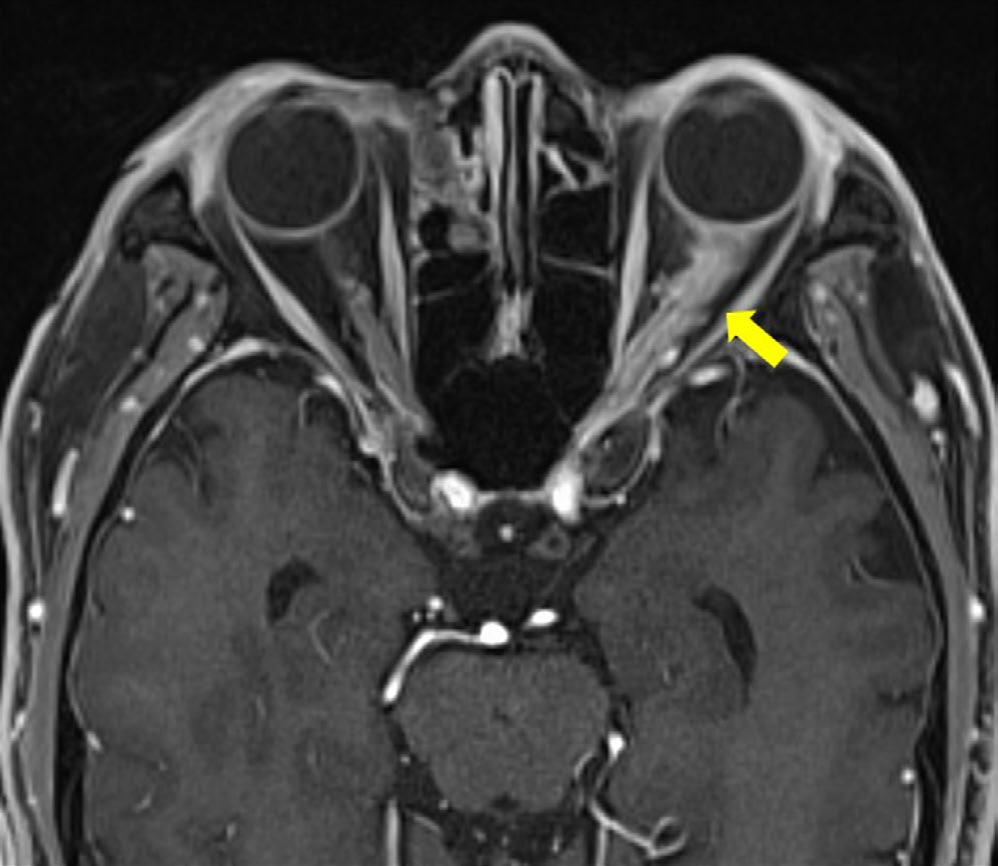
Findings of T1‐weighted orbital magnetic resonance imaging with contrast enhancement and fat suppression on admission. T1‐weighted orbital imaging with contrast enhancement and fat suppression using volumetric interpolated breath‐hold examination sequences shows swollen enhanced lesion in the left optic nerve (arrow)
